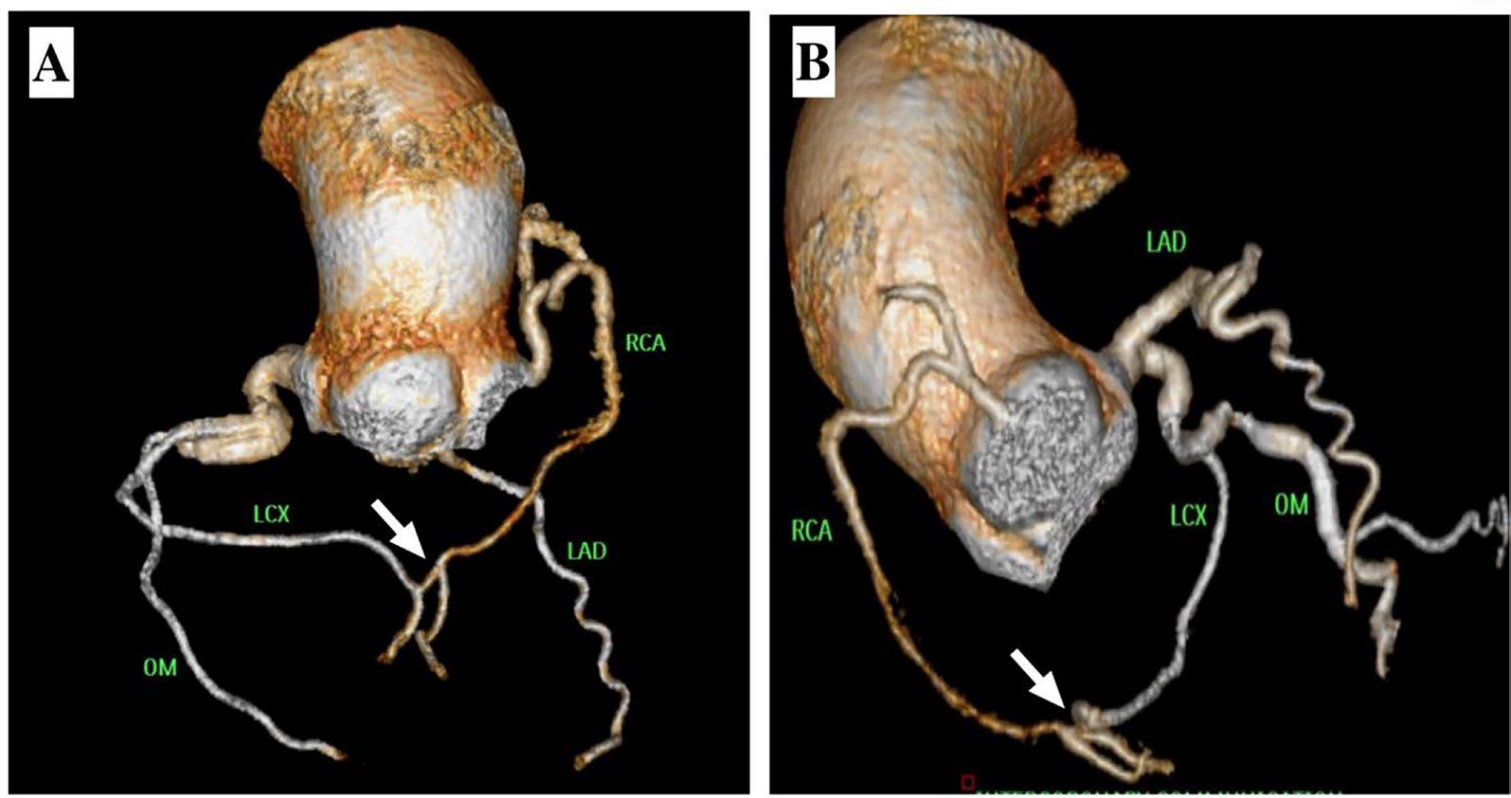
A and B Multidetector CT-coronary angiography showing an abnormal communication (white arrow) between the right posterolateral branch to the distal LCX artery
